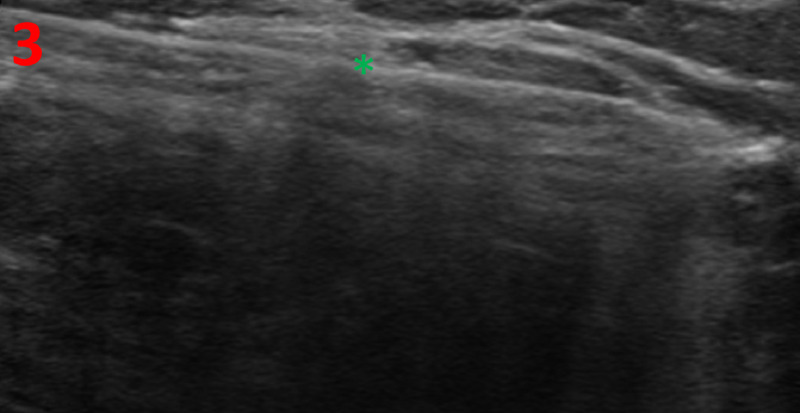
Left Breast Ultrasound-Guided Biopsy The ultrasound image shows a hyperechoic needle (green asterisk) through the mass in the upper outer quadrant at a distance of 10 cm from the nipple.
